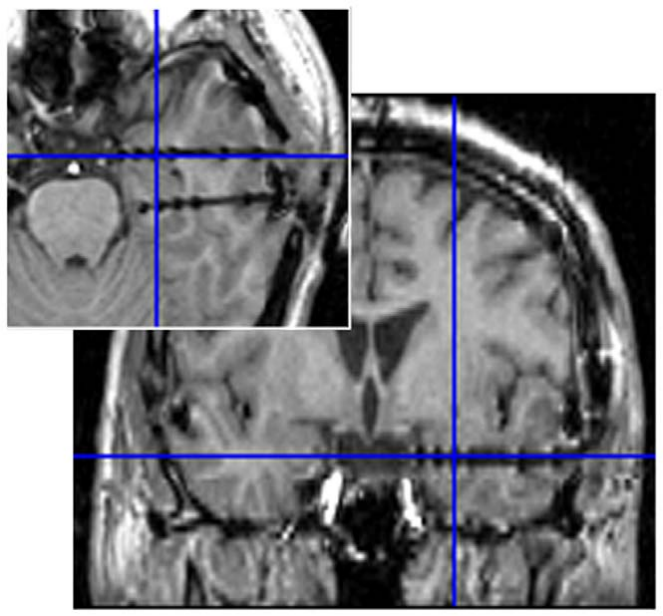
Representative anatomical magnetic resonance images. Blue crosses indicate the location of the amygdala electrodes.
